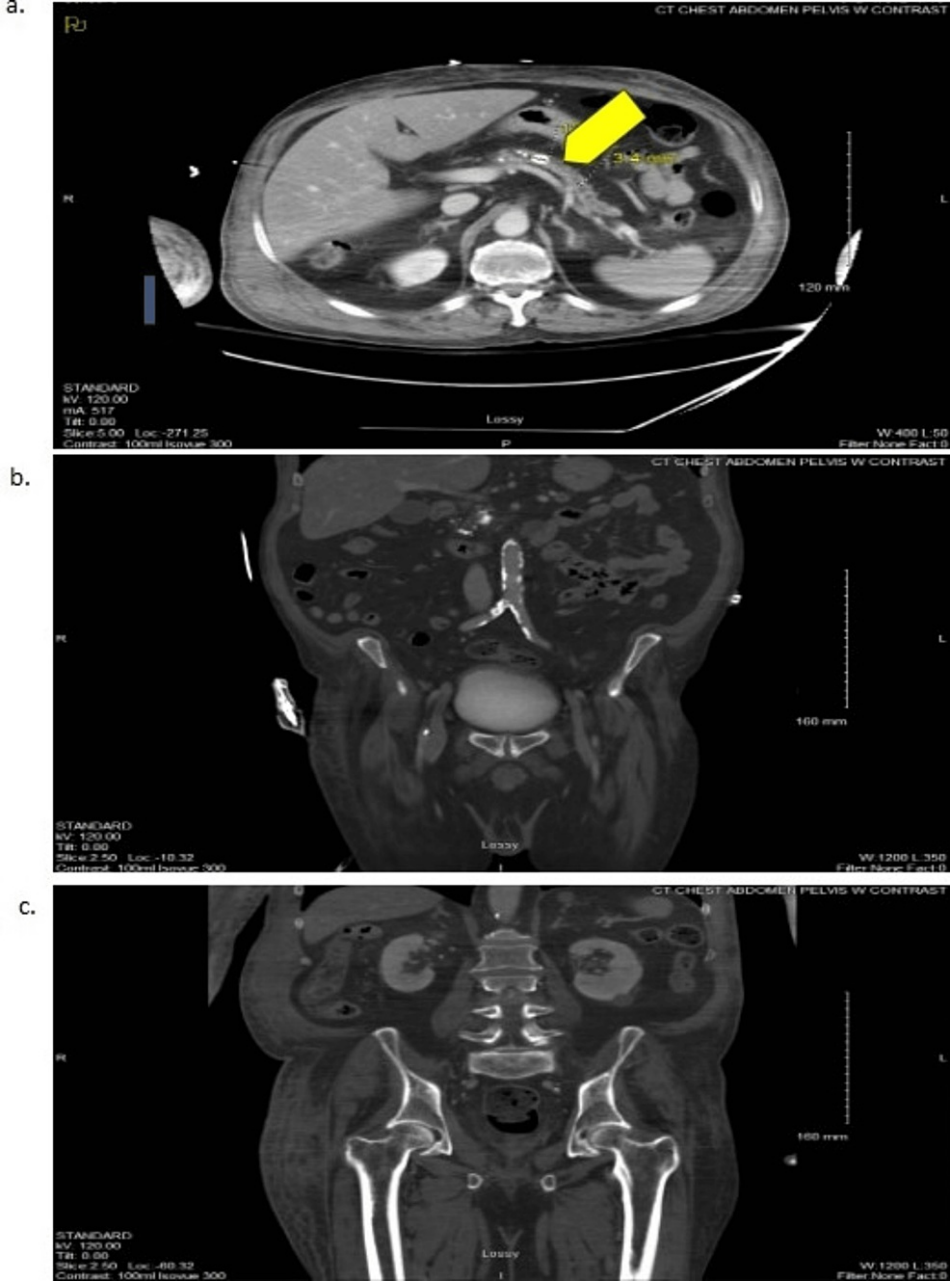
CT of the Abdomen and Pelvis With Contrast a: transverse plane, caudal (yellow arrow indicates pancreatic duct stone); b: coronal plane, caudal; c: coronal plane, rostral CT: computed tomography
